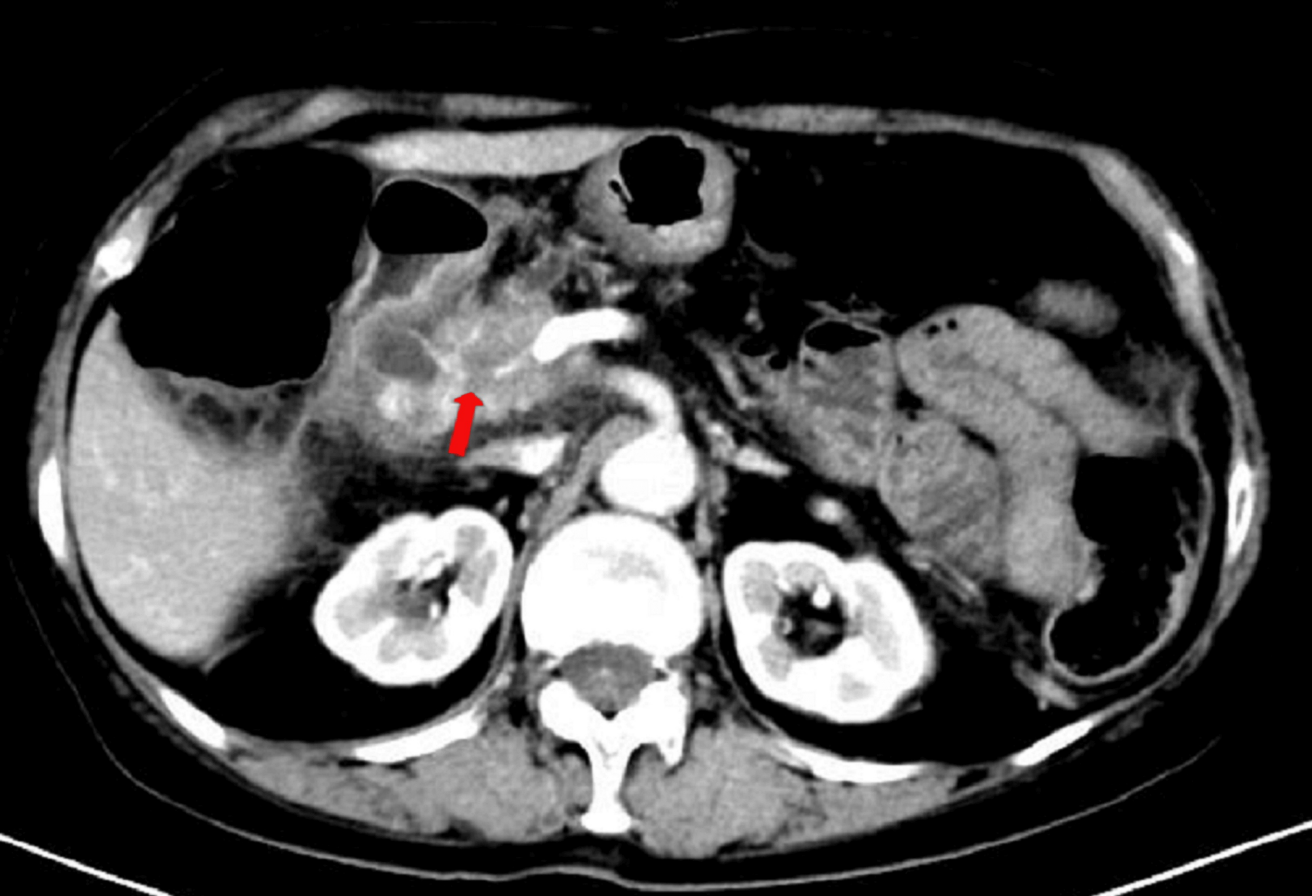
Enhanced CT on May 28, 2023, showing a soft tissue shadow in the hepatoportal-pancreatic head region with dilatation of the intrahepatic bile ducts, common bile duct, and pancreatic duct, and tumor invasion encircling the common hepatic artery and portal vein (red arrow) CT: computed tomography
